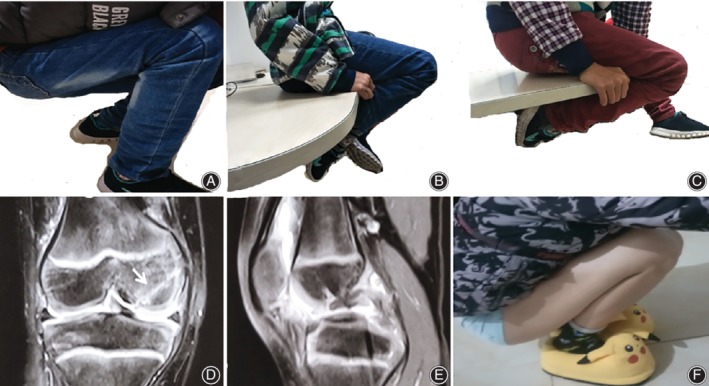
The brace was removed after 2 weeks, and full ROM was allowed at 1–month (A), 2–month (B), 3–month (C) and 24–month (F) postoperatively. The MR images obtained at 3‐month postoperatively indicate that the bone tunnel (white arrow) and the avulsion fracture are in correct positions with smooth articular surface (D, E).
